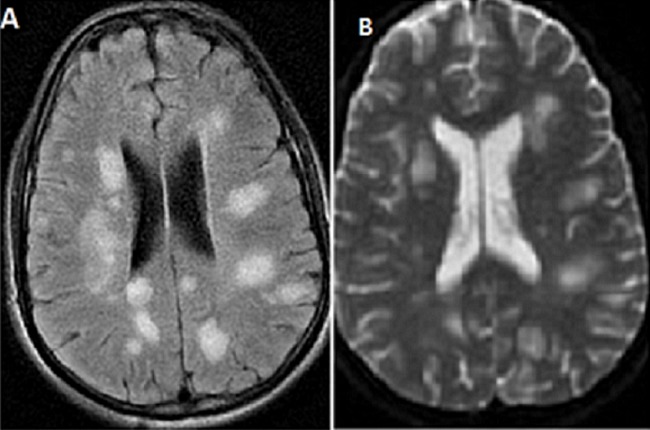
(A): IRM cérébrale séquence T2 FLAIR; (B): T2 montrant des lésions multifocales en hyper-signal de la substance blanche
